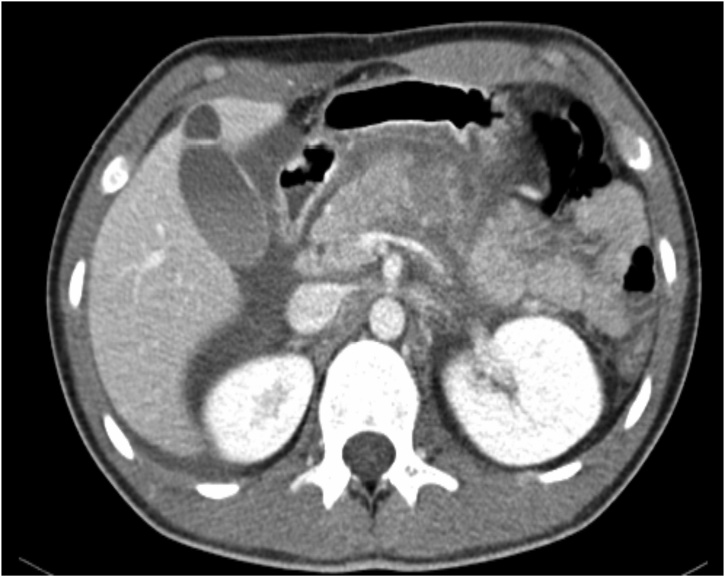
Axial view from CT abdomen & pelvis on admission - evidence of pancreatic body fracture with a pancreatic haematoma.
